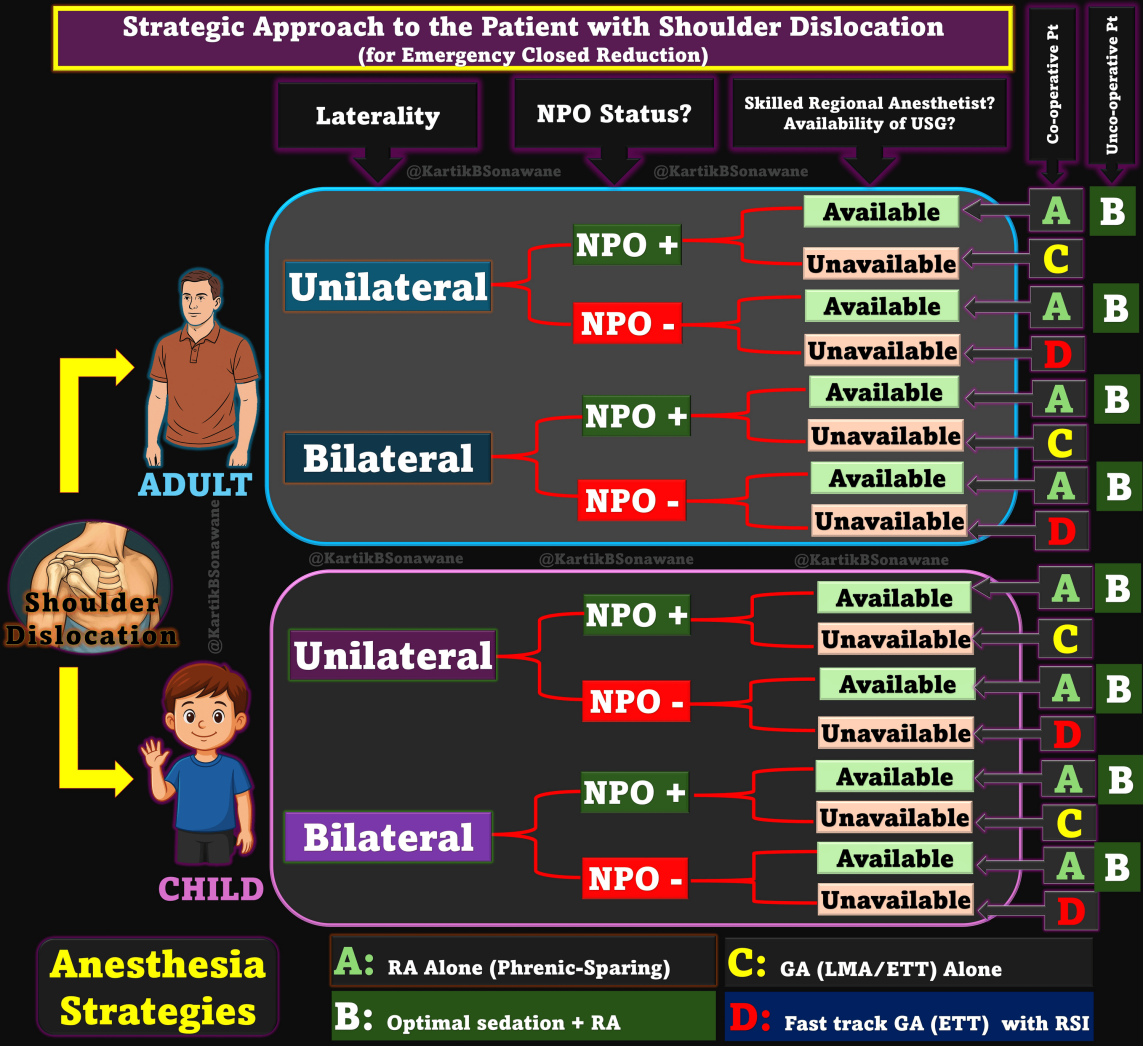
Strategic Approach to Shoulder Dislocation Management Based on Laterality and NPO Status. A clinical decision matrix for adults and children presenting with shoulder dislocations, integrating laterality (unilateral vs. bilateral), NPO status, availability of skilled regional anesthetist, and patient cooperation to guide the optimal anesthesia strategy—ranging from phrenic-sparing regional techniques to general anesthesia. NPO, nil per oral; GA, general anesthesia; RA, regional anesthesia; RSI, rapid sequence intubation; ETT, endotracheal tube; LMA, laryngeal mask airway; USG, ultrasound guidance Source: This figure was created by the author, KS.
